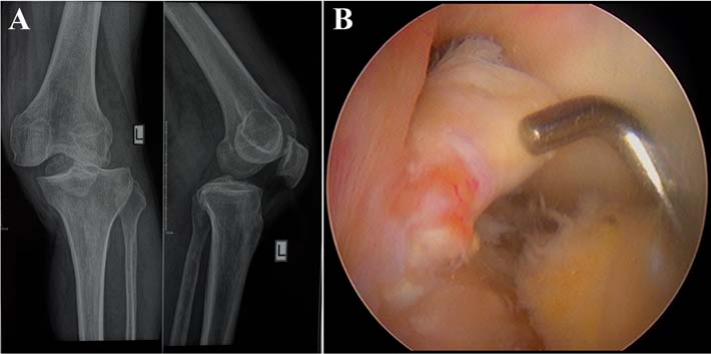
a X-ray showed the left knee dislocation; b Arthroscopy showed the anterior cruciate ligament was rupture completely through its middle (as showed by pin)
